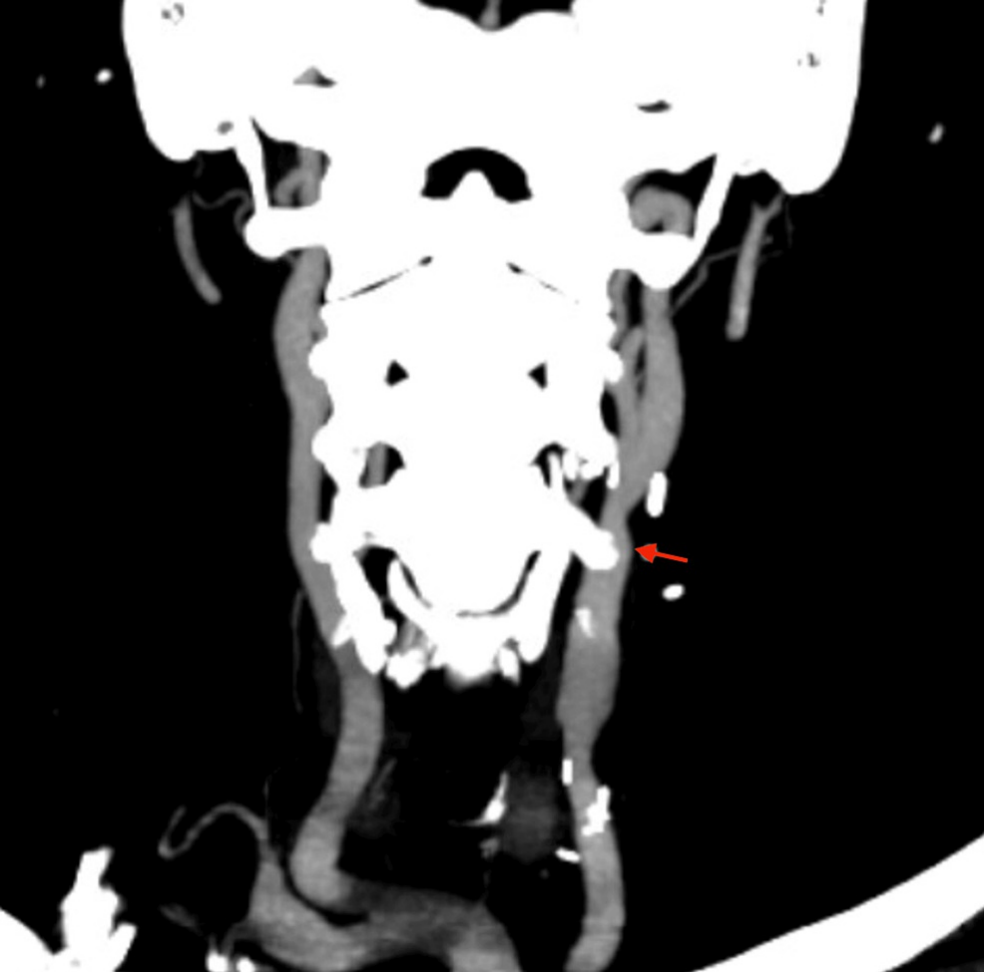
CTA showing patent left distal CCA (red arrow) after CEA. CTA: computed tomography angiography, CCA: common carotid artery, CEA: carotid endarterectomy.
